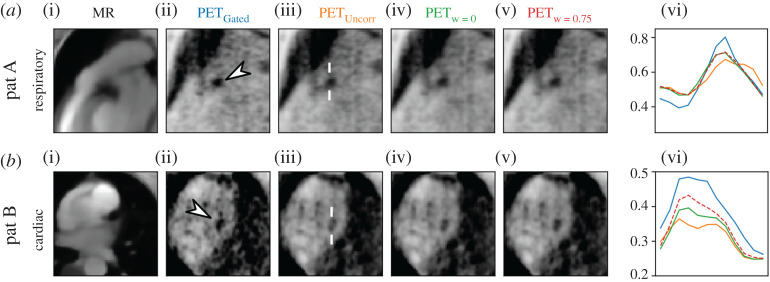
Results of patient data. Respiratory and cardiac motion-corrected PET images with different weights compared to the uncorrected (PET_uncorr_) and gated (PET_Gated_) PET images. Line plots through the plaque uptake at a location indicated by white lines in PET_uncorr_. Motion-corrected MR images are also shown as anatomical references. (Online version in colour.)
